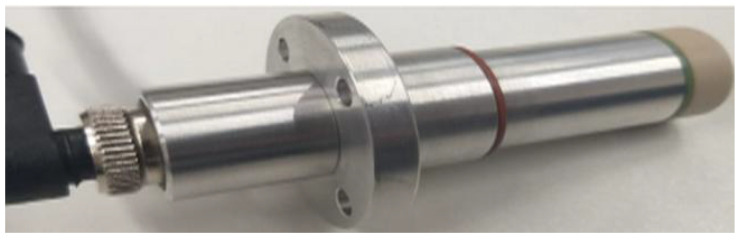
Proposed ultrasonic sensor for determination of density and flow.
